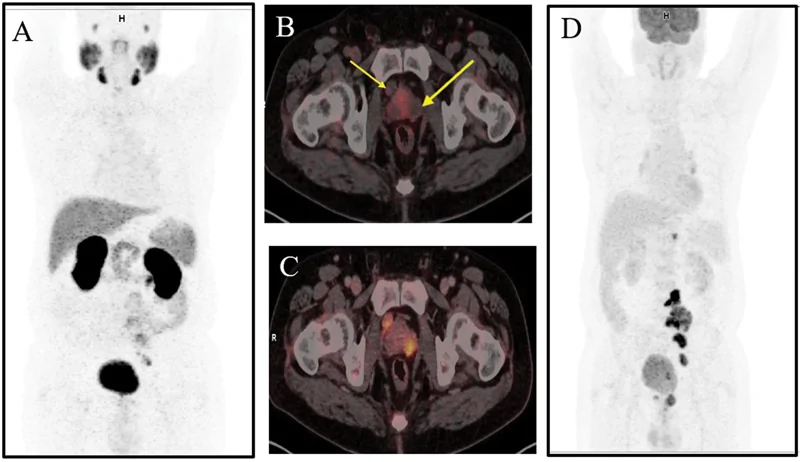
Gallium-68 (Ga-68) prostate-specific membrane antigen (PSMA) positron emission tomography/computed tomography (PET/CT). (
